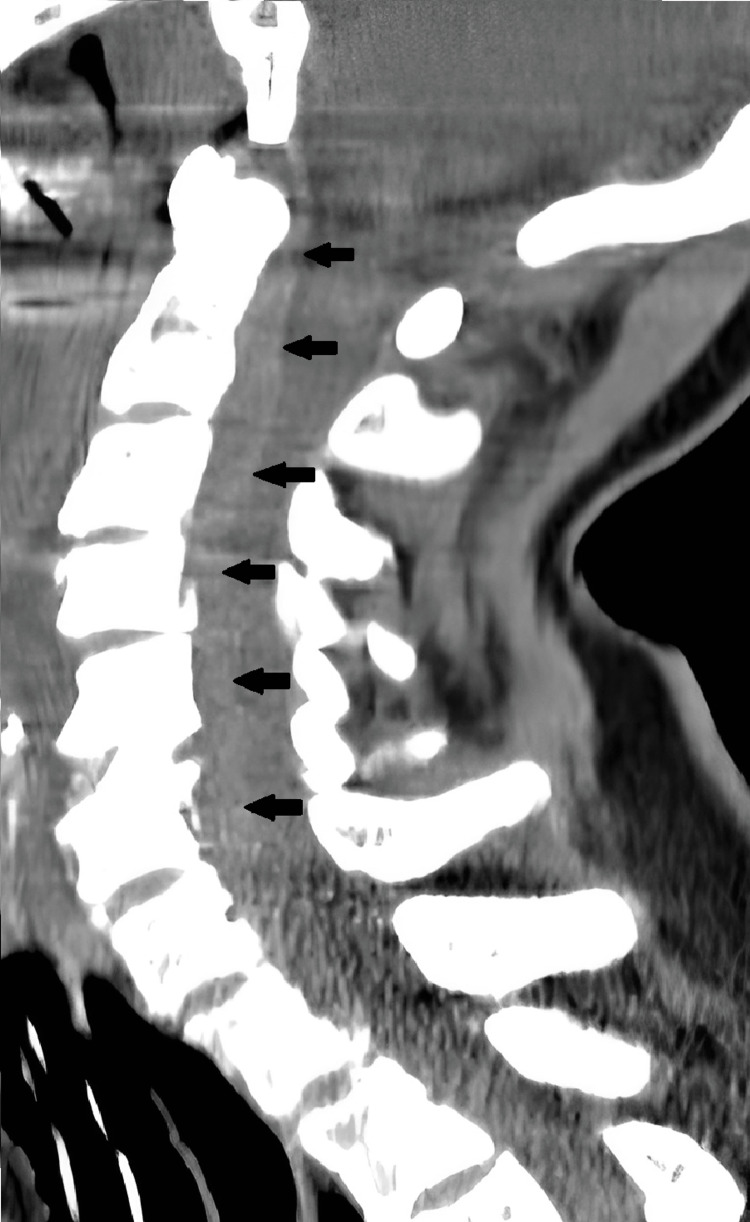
Cervical sagittal CT scan showing an anterior epidural hematoma extending from the clivus to the C6 (black arrows) and compression of the spinal cord.
